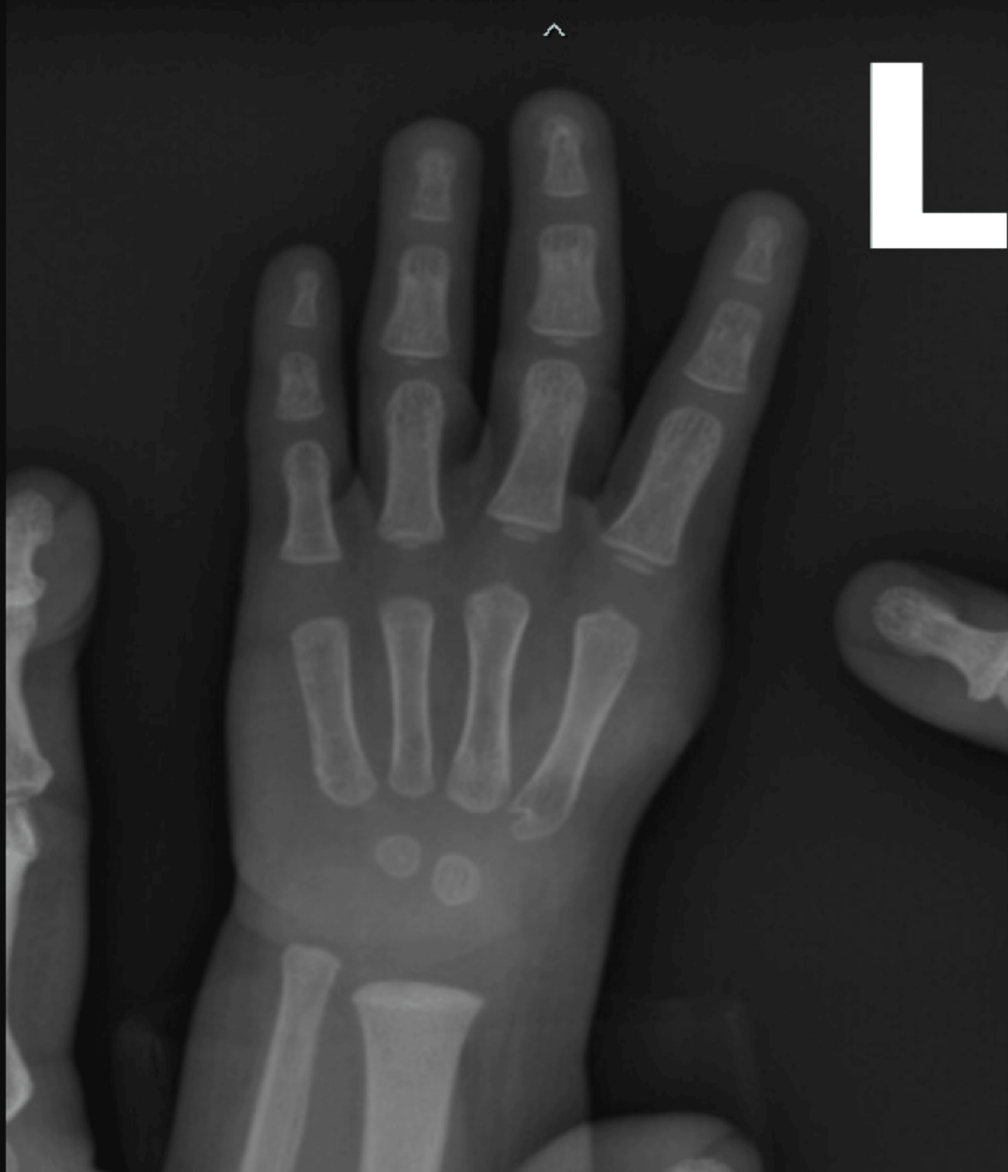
Left-hand X-ray The left thumb is absent, but the remainder of the hand is intact, and soft tissues are overall clear.
